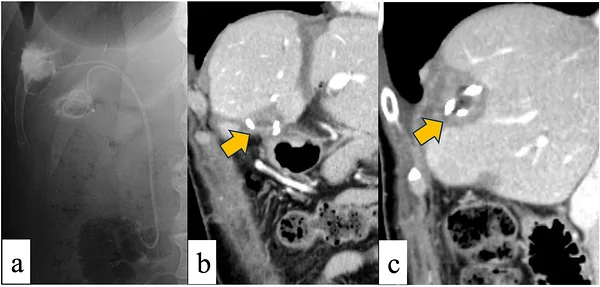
Internalization and biloma regression. (a) Fluoroscopic image during internalization on postoperative day (POD) 28: plastic stents (IYO‐stent, 5 Fr, 32 cm) were placed within each biloma cavity. (b) Coronal computed tomography (CT) after internalization: arrow indicates the markedly regressed B6 biloma. (c) CT after internalization: arrow indicates the regressed B7 biloma.
